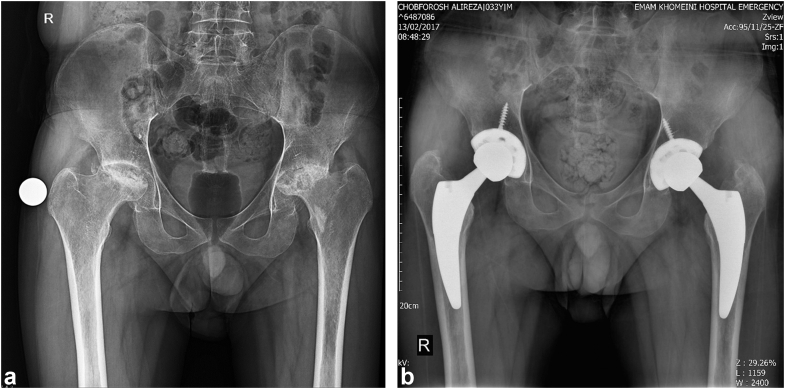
Radiographs present a case of 33-year-old patients who had bilateral avascular necrosis of femoral heads. (a) AP pelvic view shows Ficat stage IV AVN of femoral heads and Dorr type B femoral bones. (b) AP pelvic view of same patients 11 years postoperatively revealed well-fixed acetabular and femoral component. AP, anterior-posterior; AVN, avascular necrosis.
